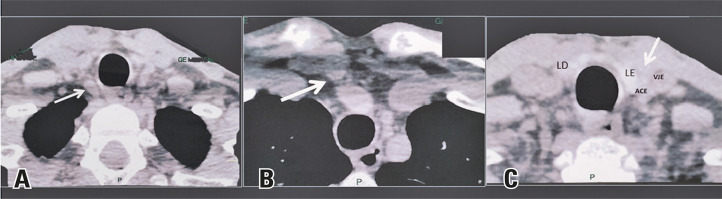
Preoperative SPECT-CT showing three possible parathyroid glands corroborating the ultrasound findings. The white arrows indicate the right superior parathyroid gland (A), right inferior parathyroid gland (B), and left superior parathyroid gland (C)
